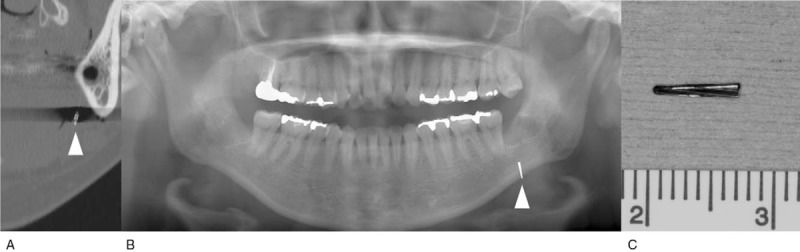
Case 2. (A) Preoperative computed tomography. (B) Preoperative panoramic radiograph. (C) The foreign body.
